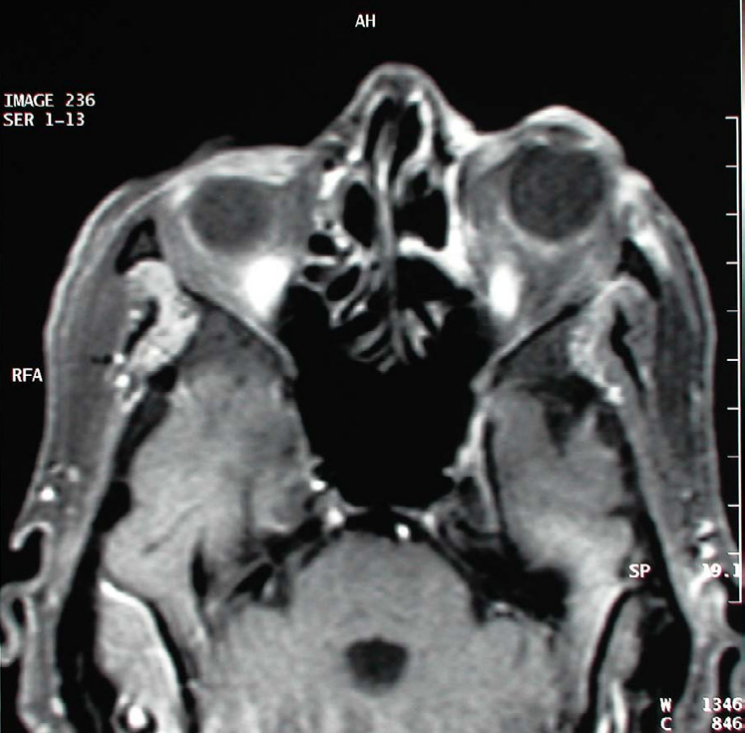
Axial magnetic resonance imaging demonstrating avert proptosis and lateral displacement of the orbit.
